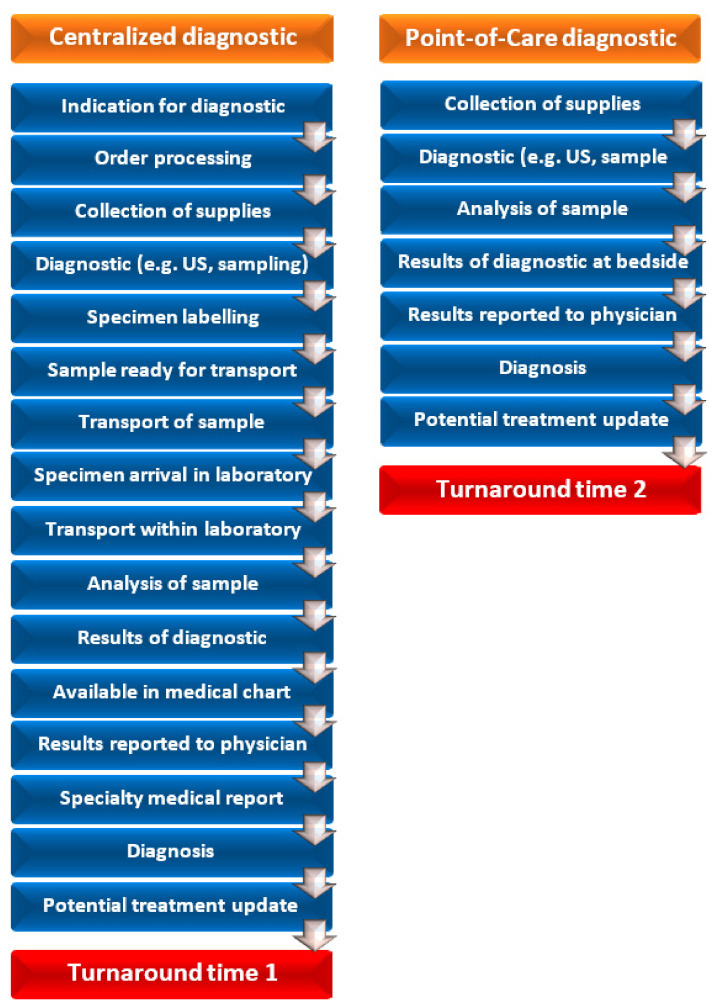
Model of activities comparison of point-of-care and central centralized diagnostics. US reflects ultrasound.
